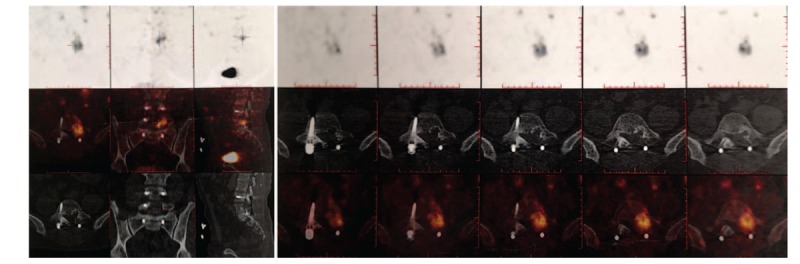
Positron emission tomography-computed tomography revealed the recurrent pathogenic tumor at L5.
